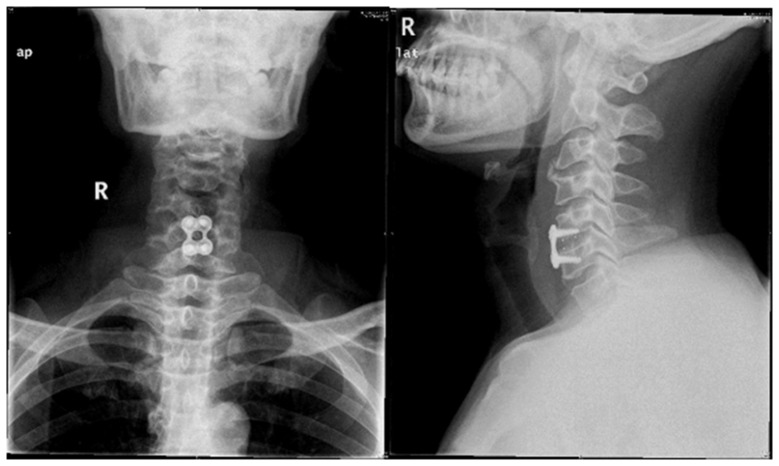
Case 1. X-ray examination three months subsequent to the C5–6 anterior decompression-fusion and internal fixation surgery.
